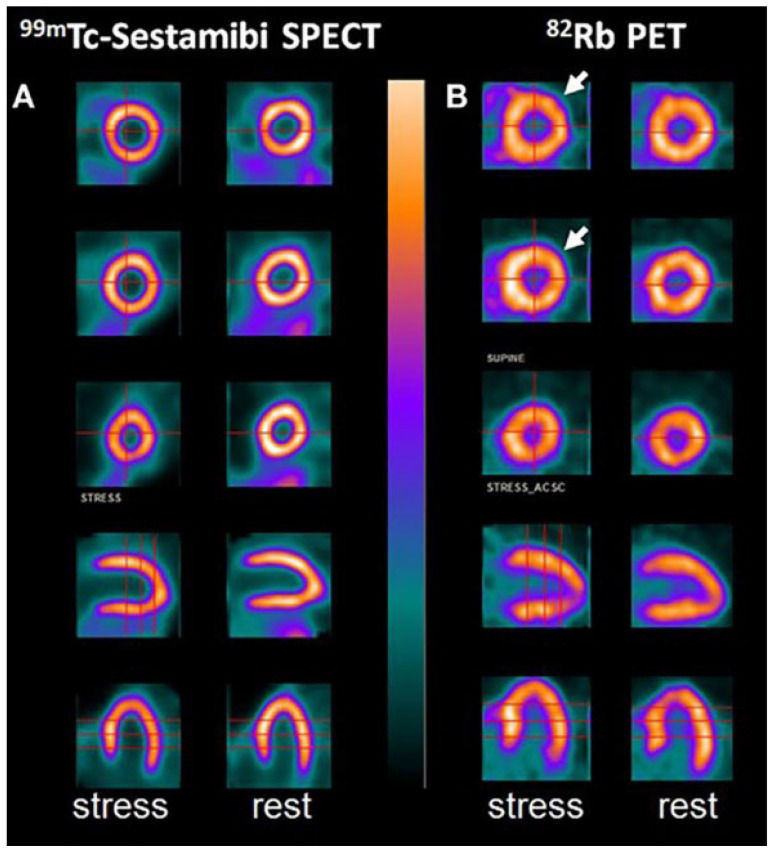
(A) ^99m^Tc-Sestamibi SPECT images in both stress and rest state. (B) ^82^Rb PET images in both stress and rest state. ^82^Rb-PET images depict ischemia involving the circumflex artery (arrow), shown after dipyridamole administration. ^99m^Tc = radioactive isotope technetium-99 m; SPECT = single-photon emission computed tomography; ^82^Rb = radioactive isotope rubidium-82; PET = positron emission tomography. Source: Chatal, J.F., Rouzet, F.; Haddad, F.; Bourdeau, C.; Mathieu, C.; Le Guludec, D. Story of rubidium-82 and advantages for myocardial perfusion PET imaging. *Front. Med.* 2015, *2*, 65.
